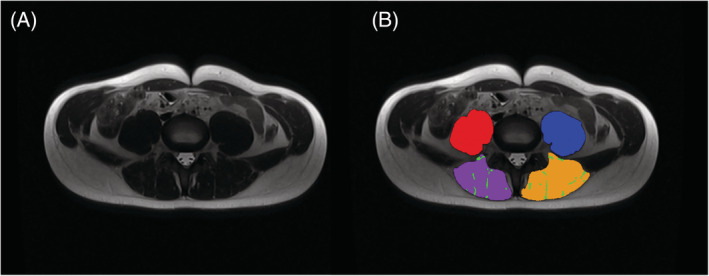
A 17‐year‐old boy (BMI: 19.4 kg/m^2^). (A) Native MRI T2‐weighted sequences in the transverse plane at the level of L4/L5 intervertebral disc. (B) Example of tissue segmentation at the same level: Right PMA (red), left PMA (blue), right PSMA (purple), left PSMA (yellow) and tMFI (green). BMI, body mass index; PMA, psoas muscle area; PSMA, paraspinal muscle area; tMFI, total macroscopic fat infiltrations into PSMA.
